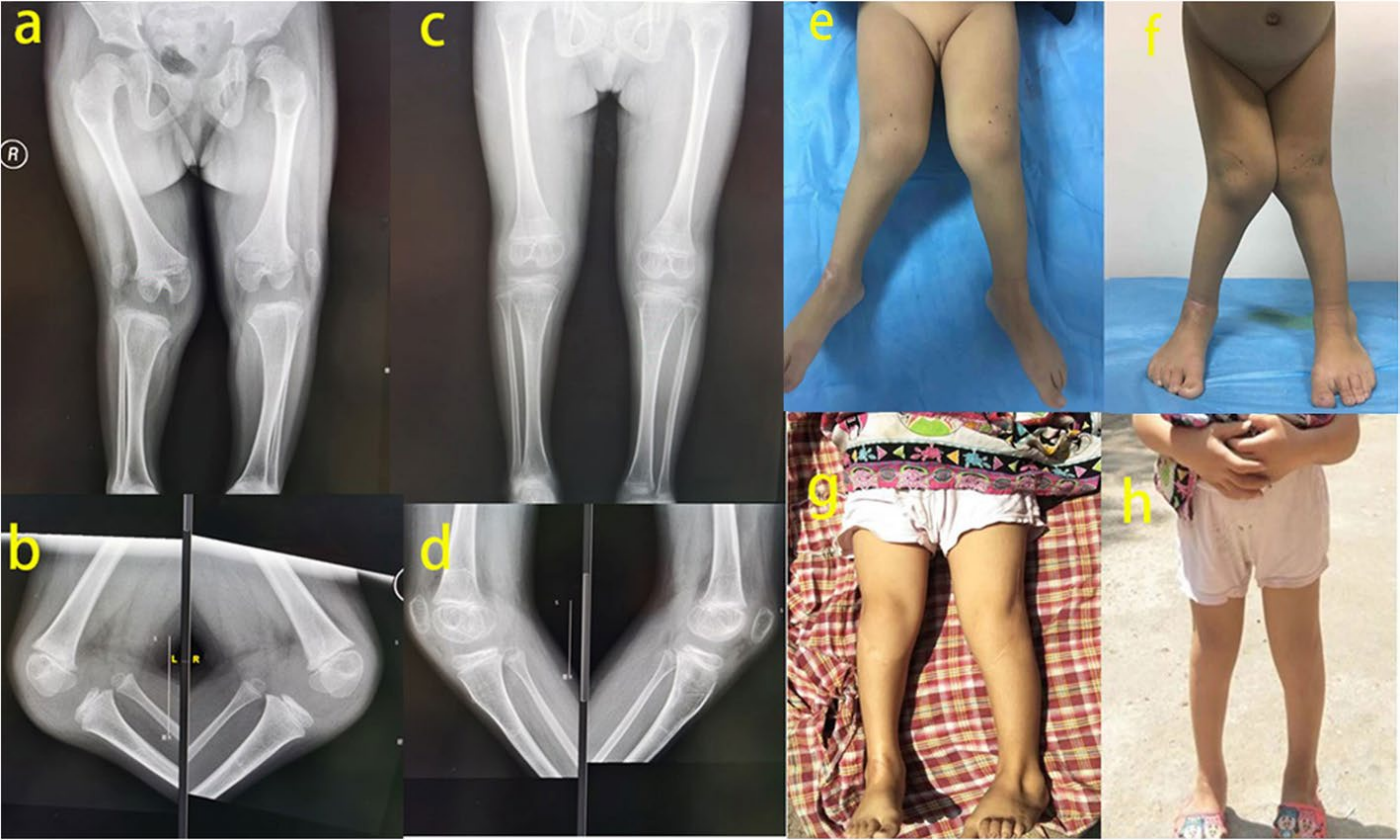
Preoperative and postoperative radiographic and Appearance manifestations. a, b X-rays before the operation indicated bilateral lateral dislocation of the patella and genu valgum of the right knee. c, d Eighteen months after the operation, genu valgum improved significantly on X-rays. e, f, g, h Appearance of the lower extremity pre- and postoperation
